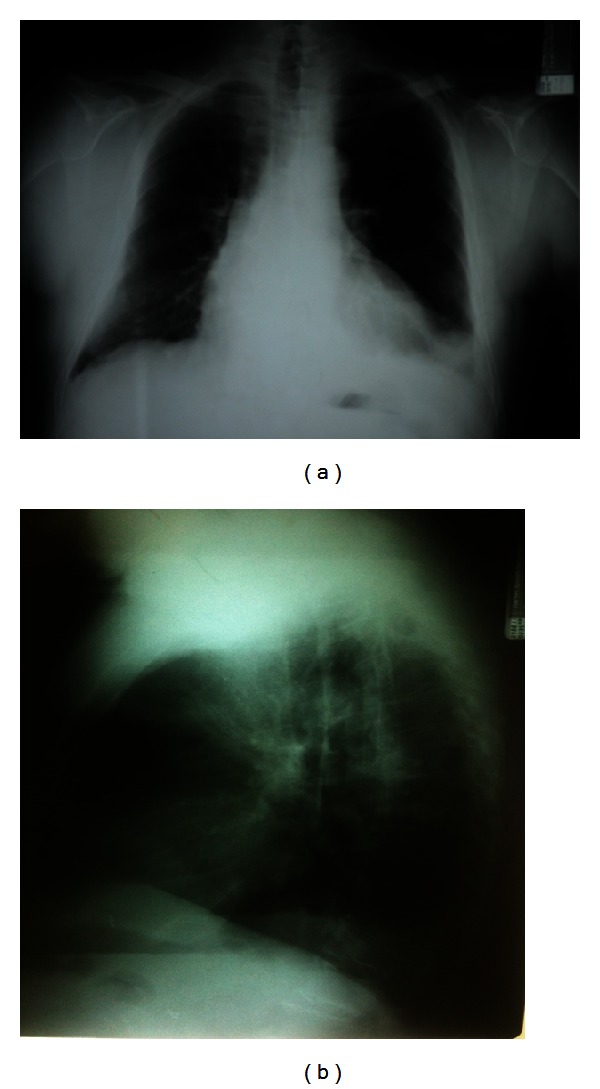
Chest X-ray of the patient on admission in the nephrology department.
